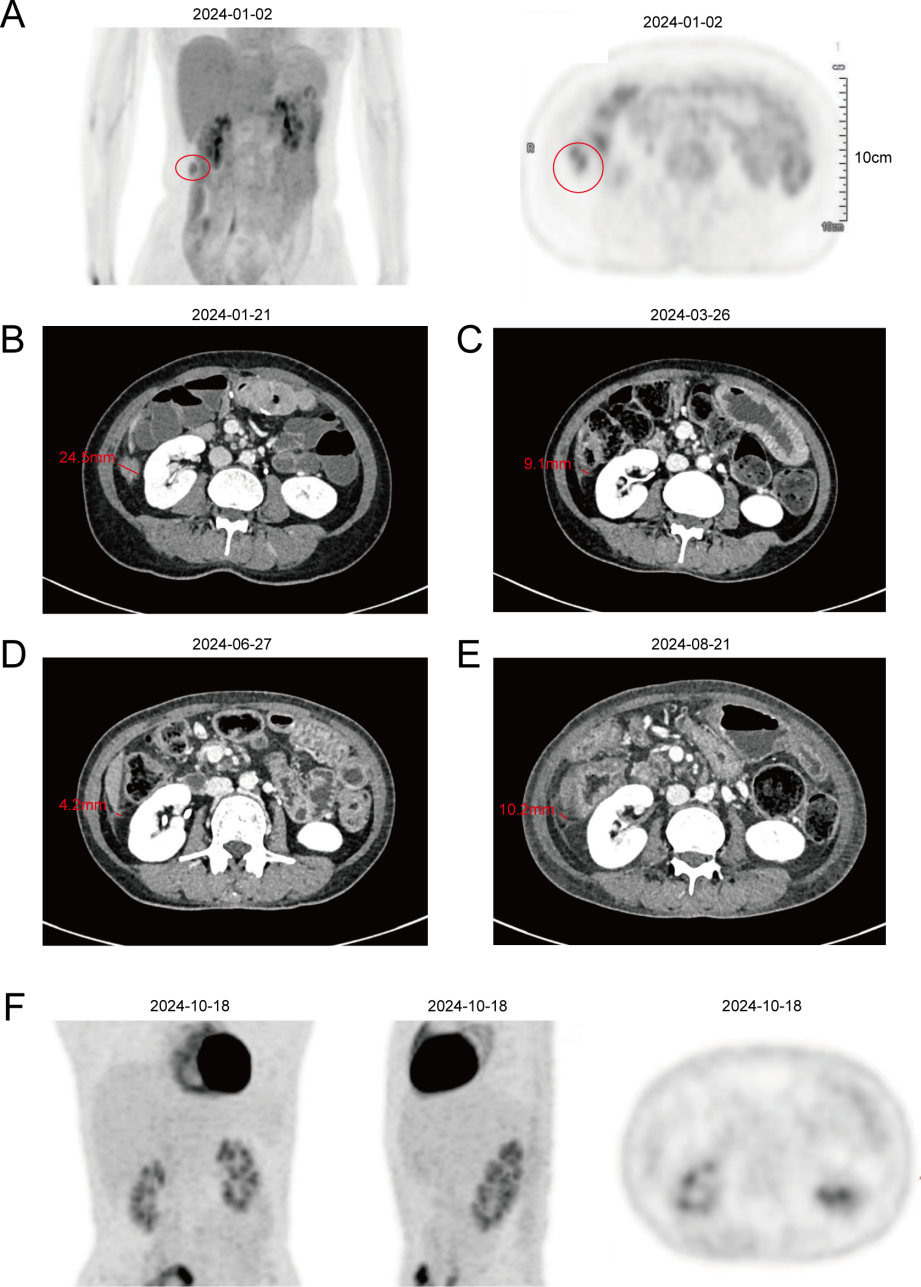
Imaging images of the patient after peritoneal metastasis. (A) PET/MR images of the patient. (B–E) CT images of the patient. (F) PET/CT images of the patient.
